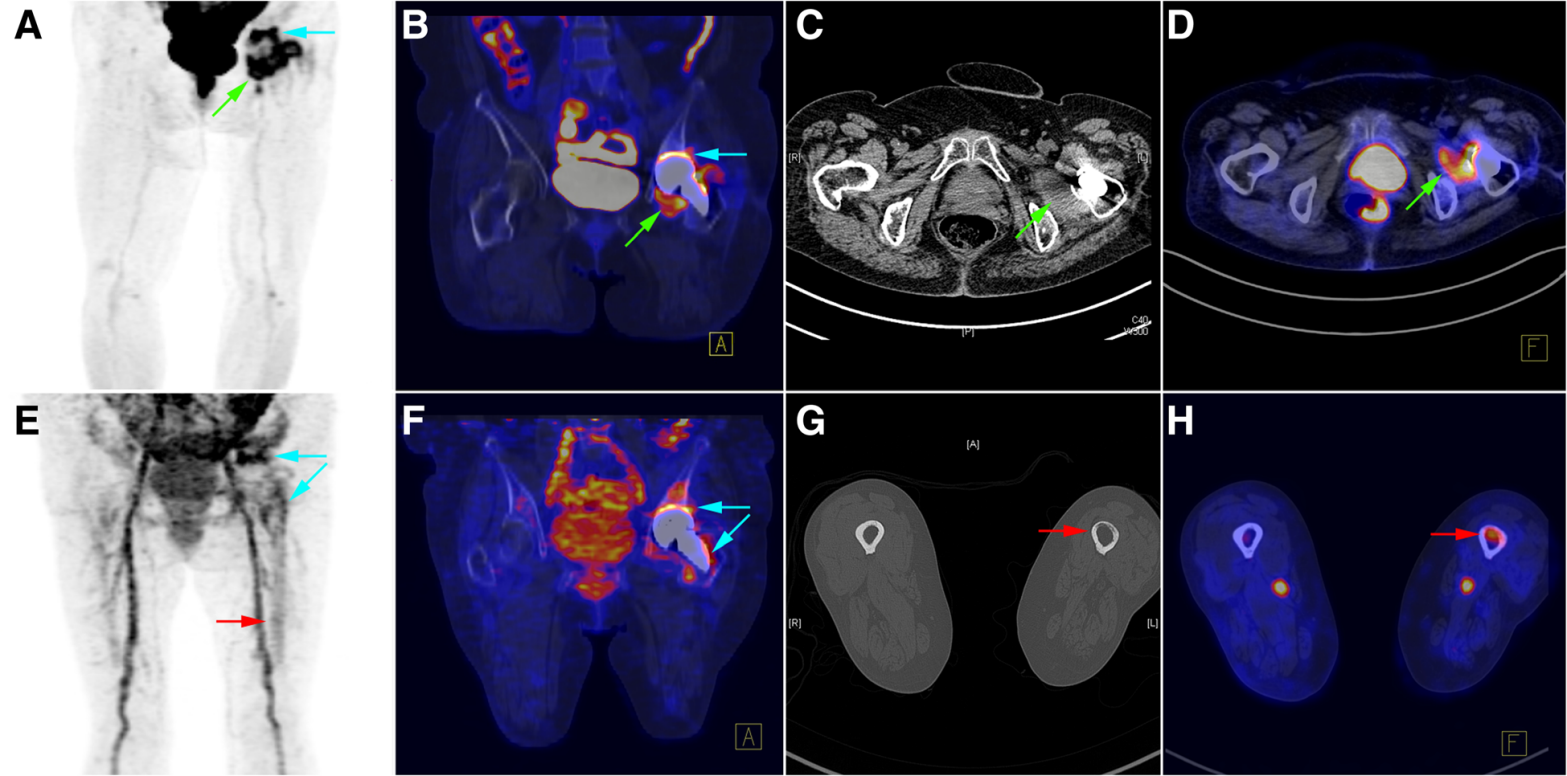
Patient (case # 14) with a history of hip prosthesis implantation performed 8 months before imaging. The results of ^18^F-FDG PET/CT (upper row) and ^68^Ga-citrate PET/CT (lower row) are presented. a–d The green arrows indicate an increased ^18^F-FDG uptake occurring in necrotic tissue located within a swollen left quadratus femoris muscle. There was also evidence of an increased ^18^F-FDG uptake occurring at the bone-prosthesis interface (cup component; blue arrow). Taken together, these findings were suggestive of an infectious process which was confirmed intraoperatively (presence of cloudy synovial fluid and necrotic tissue). e–h The blue arrows indicate an increased ^68^Ga-citrate uptake occurring at the bone-prosthesis interface (especially in the proximal portion). An osteolytic change in the anterior cortex of the left femur was observed on a CT image, accompanied by an increased ^68^Ga-citrate uptake (which was present in the adjacent bone marrow as well, red arrows)
